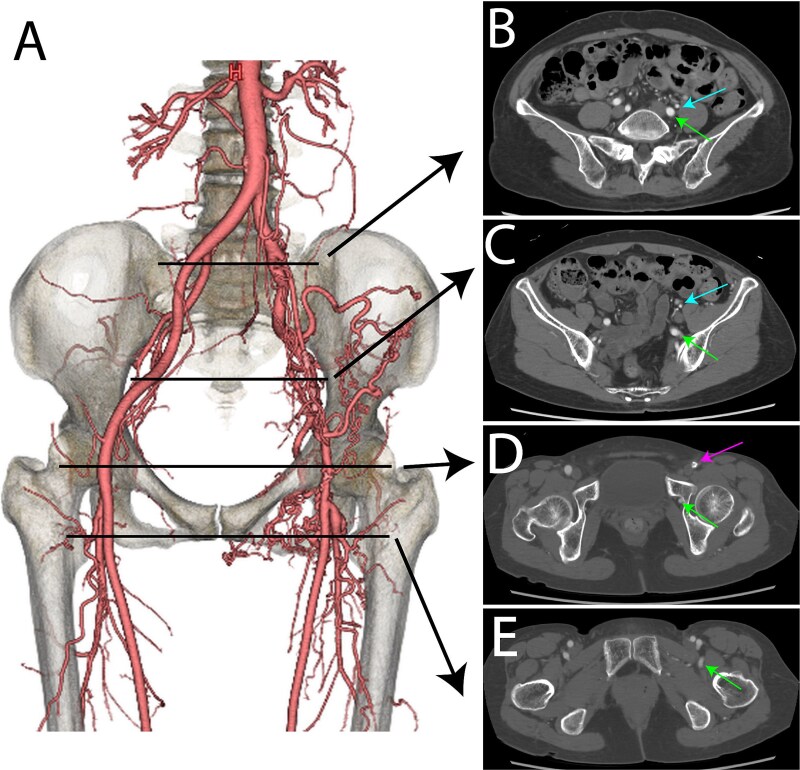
Preoperative contrast-enhanced CT images. (A) Volume-rendered (VR) image. (B) Bifurcation level of the external iliac artery (blue arrow) and the internal iliac artery. The sciatic artery is marked with a green arrow. (C) Pelvic level. The external iliac artery (blue arrow) and the sciatic artery (green arrow) are shown. (D) Femoral head level. The left common femoral artery (pink arrow) and the sciatic artery (green arrow) are displayed. (E) Postbifurcation of the superficial femoral artery and deep femoral artery. The sciatic artery (green arrow) is visible.
